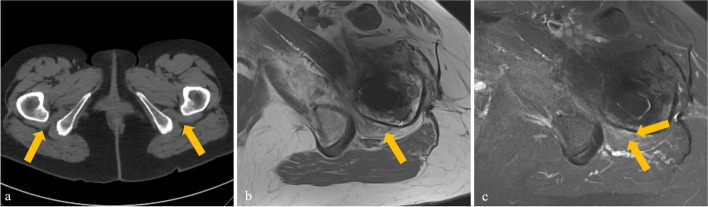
A 72-year-old female patient admitted for buttock pain following THA with clinical suspicion of IFI. Preoperative CT imaging depicting no relevant narrowing of the left ischiofemoral space compared to the contralateral side (arrows) or fatty atrophy of the quadratus femoris muscle (a). Postoperative axial T1-weighted MR image shows fatty atrophy of the quadratus femoris muscle (arrow) and narrowing of the ischiofemoral space (b). The corresponding axial STIR image reveals an edema of the quadratus femoris muscle (c, arrows). IFI, ischiofemoral impingement; STIR, short-tau inversion recovery; THA, total hip arthroplasty
